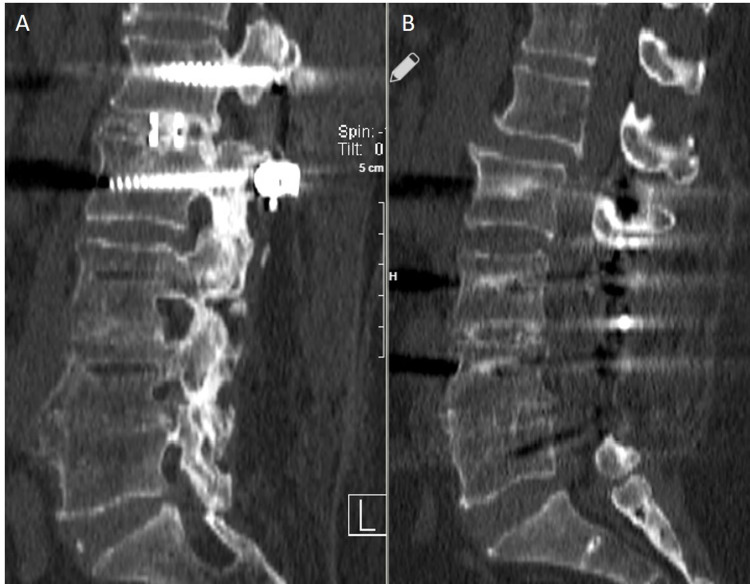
A: Postoperative 2nd Year CT, B: Preoperative CT A: Posterior transpeduncular stabilization and TLIF fusion are seen at the L1-L2 level. B: It is seen that the patient with an adjacent segment has instability at the L1-L2 level.
